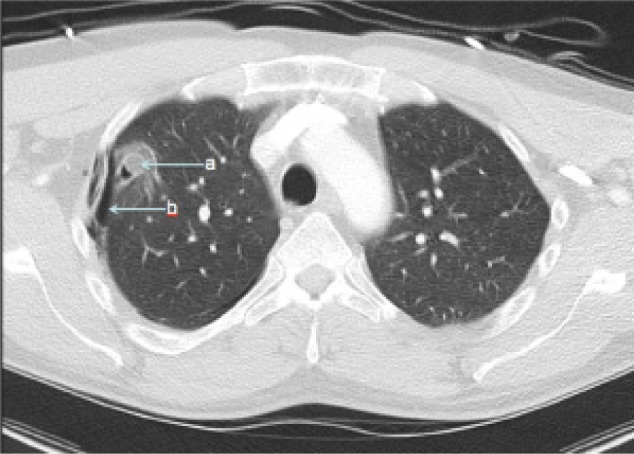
Computed tomography of thorax showing: a) stick and b) pneumothorax
